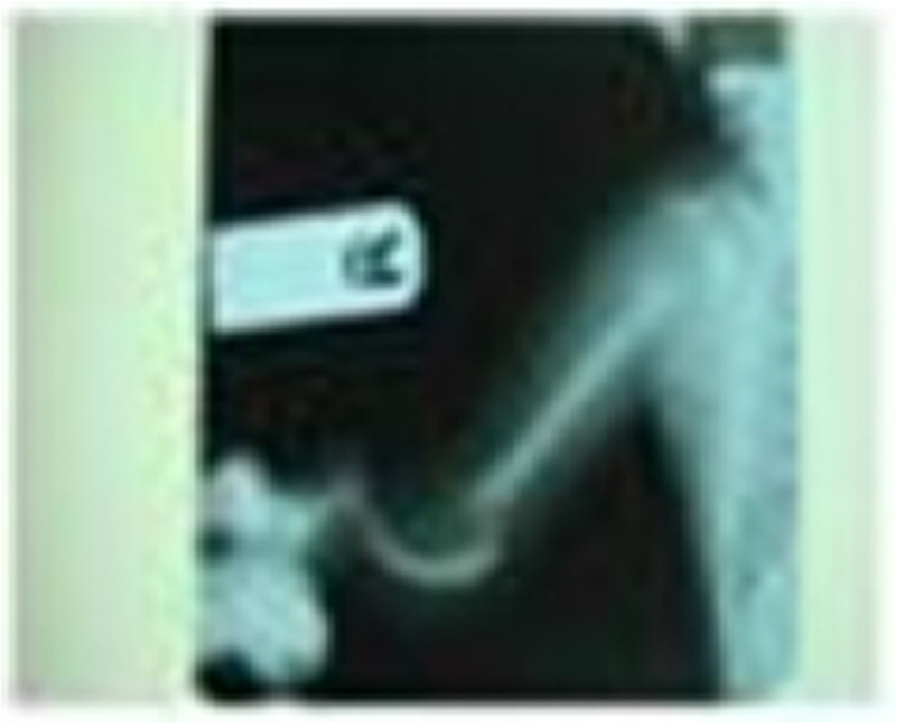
Plain X-ray of the right upper limb
